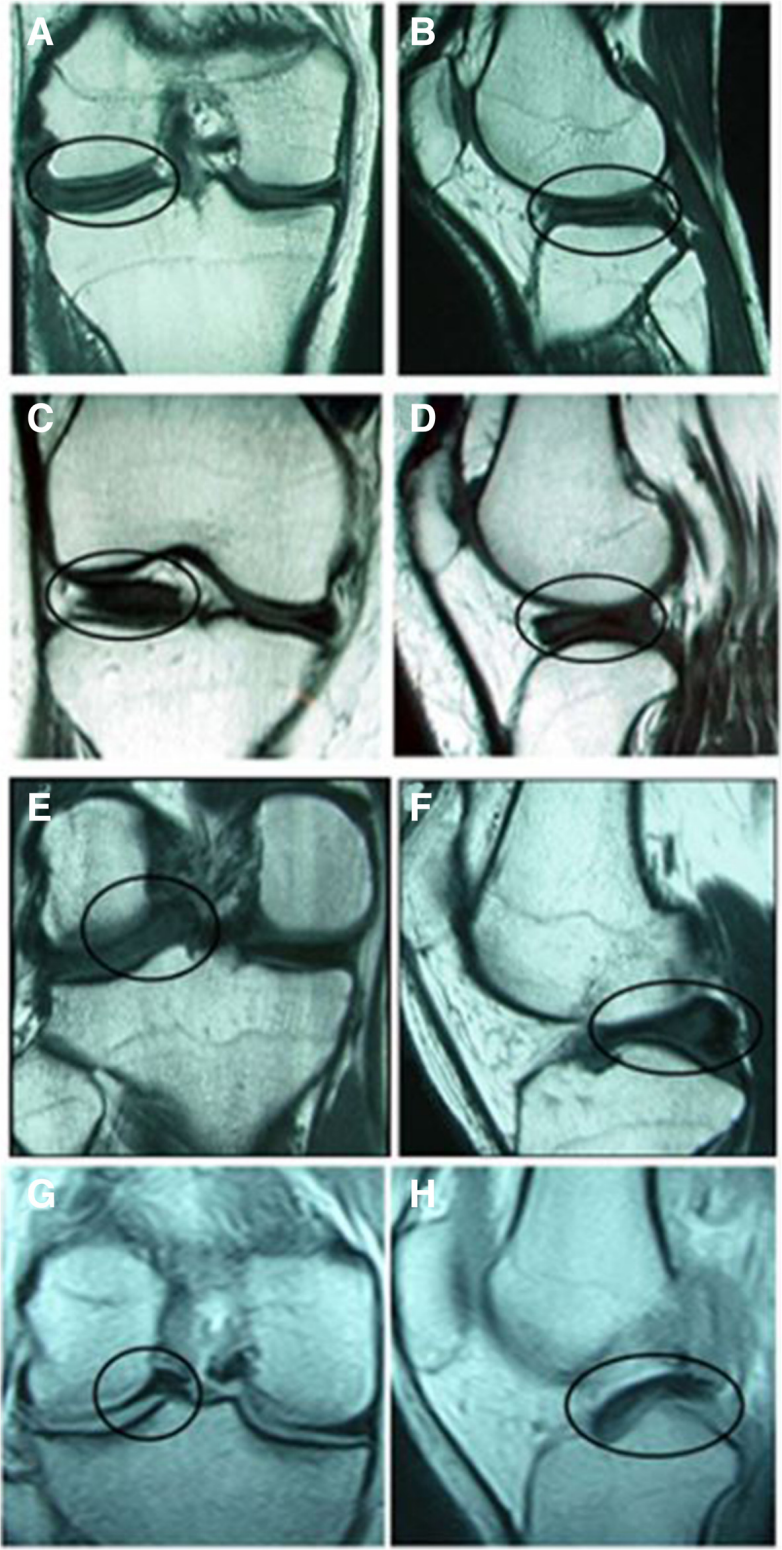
T1-weighted MR images of DLM with different types of shifts. Coronal (a) and sagittal (b) images of no shift, coronal (c) and sagittal (d) images of anterocentral shift, coronal (e) and sagittal (f) images of posterocentral shift and coronal (g) and sagittal (h) images of central shift
